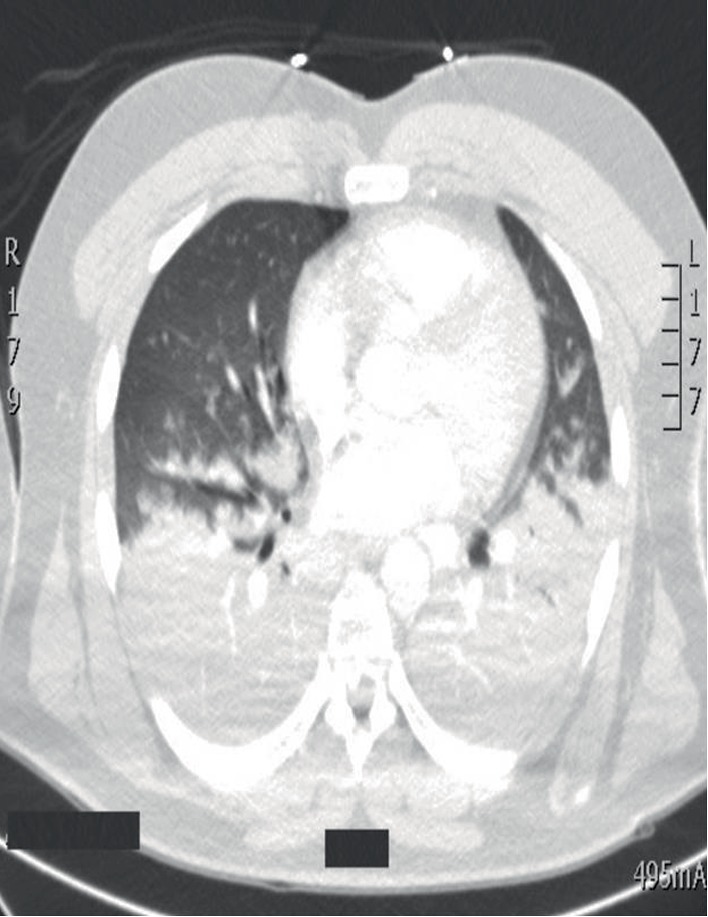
CT chest (axial view) demonstrating bilateral lung infiltrates, more prominent in the lower lobes.
